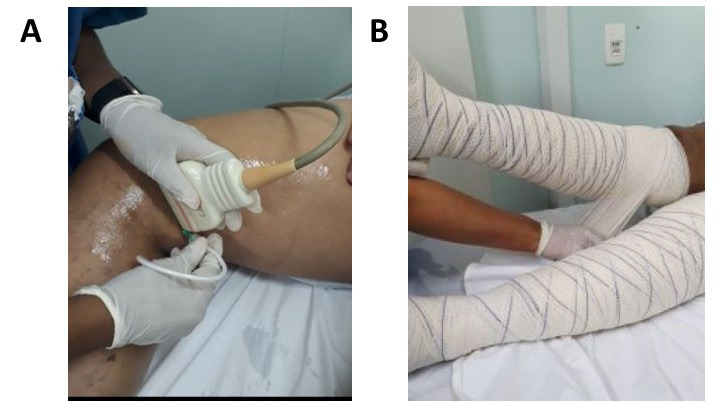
(A) Ultrasound-guided puncture and (B) compressive bandaging.
